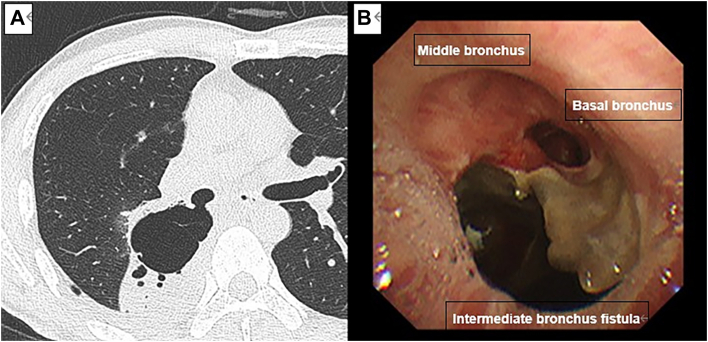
(A) Computed tomography and (B) bronchoscopy show the intermediate bronchus fistula.
